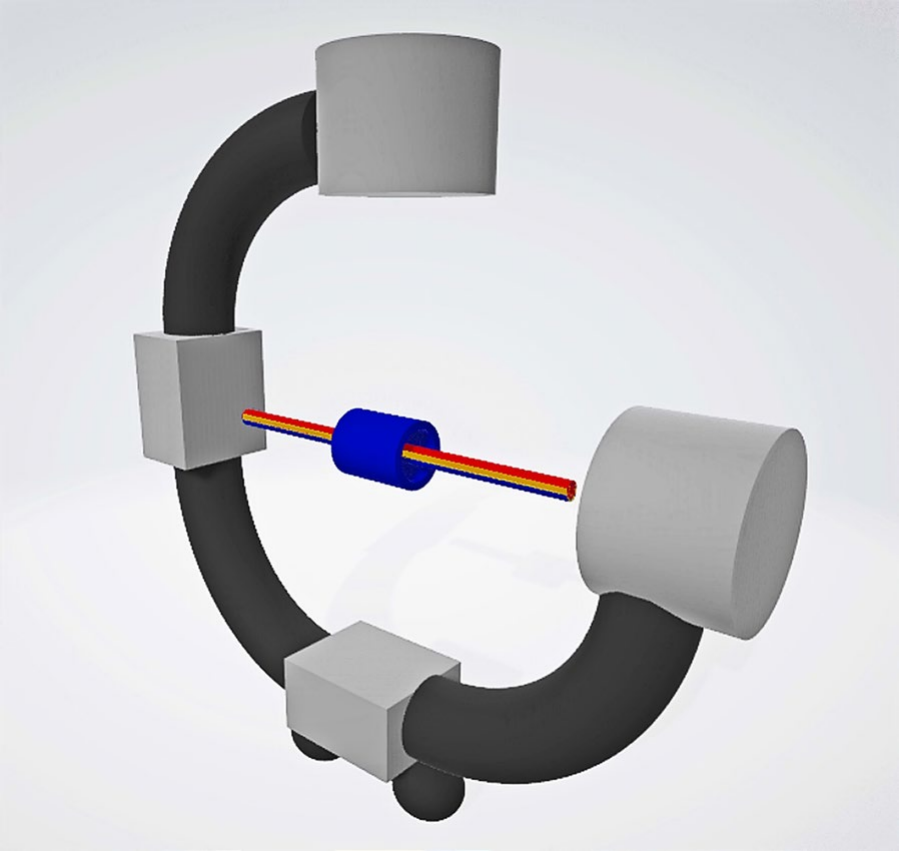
Work principle sketch maps of the device. This meant that the X-ray central fluoroscopy axis (the yellow line), the two laser lights cross axis (the red line) and the target screw passage (the blue line) remain coaxial, known as “three-line coaxial”. The blue tube represented a nail hole
